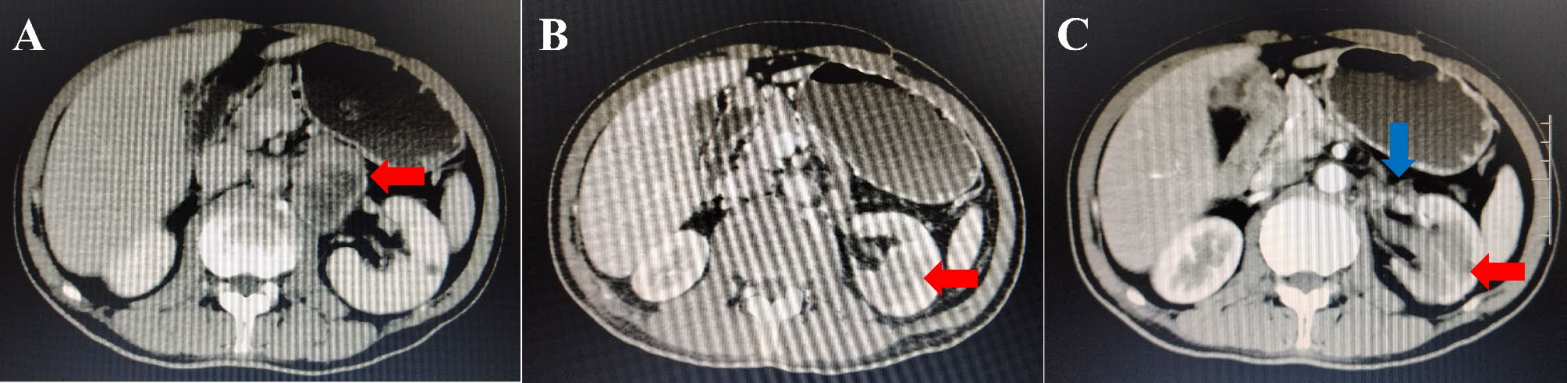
A. Contrast-enhanced CT shows a tuberculous retroperitoneal mass (red arrow) on the left side of the kidney. B. 3 months later, at the first abdominal CT review, a mass about 2.5 cm in size was found occupying the left hilum (red arrow). C. 5 months later, the second abdominal CT review revealed a large and small 3.6 cm soft tissue mass under the left kidney (red arrow) and multiple soft tissue shadows in the renal portal area (blue arrow)
